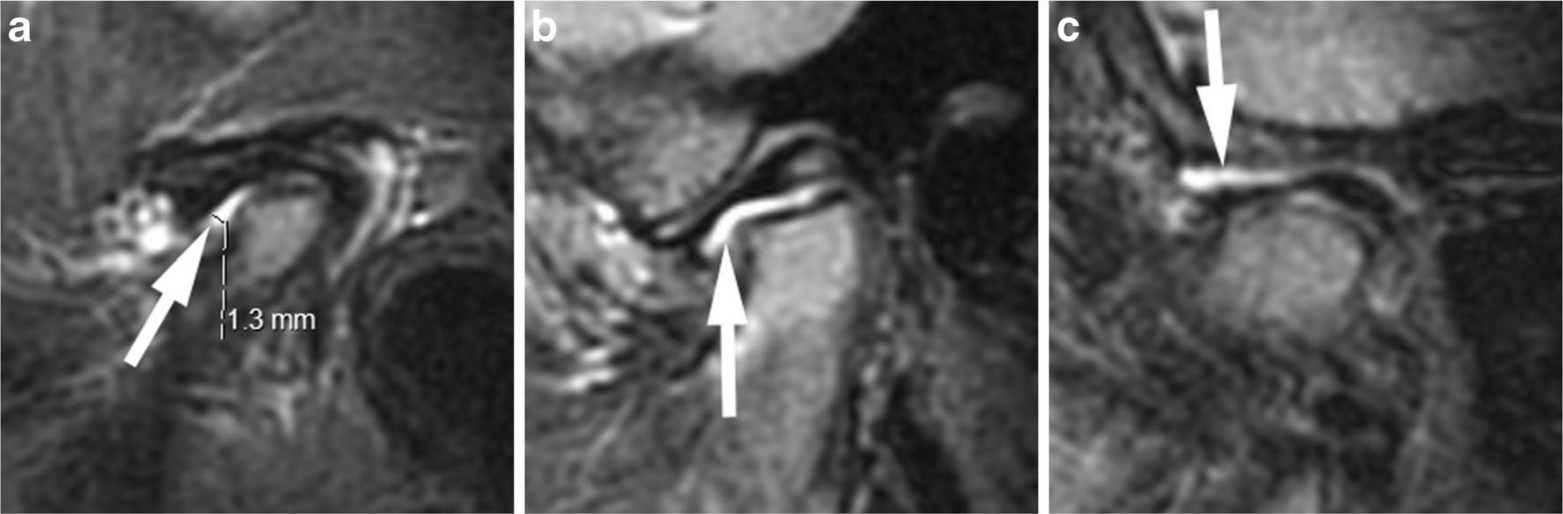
Small joint effusion (grade 1). a-c Sagittal oblique fat-saturated T2-weighted fast spin echo images (TR/TE 5,400/77 ms) show high signal intensity (arrows) of >1-mm and ≤2-mm thickness within the joint spaces in the lower anterior recess in a 6-year-old girl (a), in the lower joint compartment (b) and upper joint compartment (c) in a 5-year-old boy. Note mild flattening of condyle in (a), and moderate/severe flattening of the condyle in (b) and (c)
